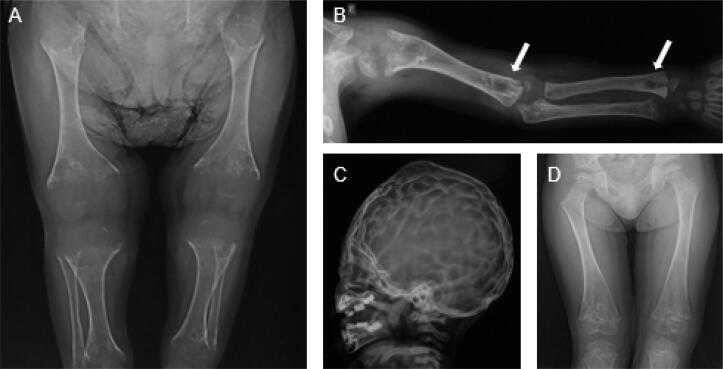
Male patient with a benign prenatal form of hypophosphatasia. He had presented manifestations before birth but was only diagnosed with the disease at the age of 1 year and 8 months. He was started on asfotase alfa at the age of 2 years and 10 months. (A) Age 1 year and 2 months: upper limb radiograph showing diffuse bone hypomineralization, cupping, fraying, and widening metaphyses, thin cortical bone, and diaphyseal shortening. (B) Metaphyseal radiolucent “tongues” (arrows). (C) “Beaten copper” sign. (D) Age 5 years, after 2 years of treatment, showing improved bone mineralization and formation, cupping, fraying and widening metaphyses, and radiolucent “tongues.” The long bone became better defined, and the lytic and sclerotic metaphyseal areas resolved. Adapted from: Arch Endocrinol Metab. 2021;64(5):623-9.
